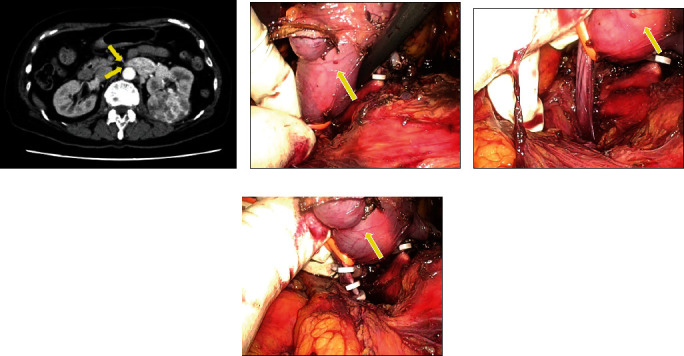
Imaging and intraoperative findings of the cases with left renal cell carcinoma with thrombus (case 3). (a) Computed tomography demonstrated a thrombus extending 2 mm beyond the right aortic border. (b) A vessel loop hooked to the thrombus tip. (c) The thrombus was pulled up using a vessel loop. (d) The left renal vein was dissected using Hem-o-lok clips. Arrowheads indicate the tip of the tumor thrombus.
